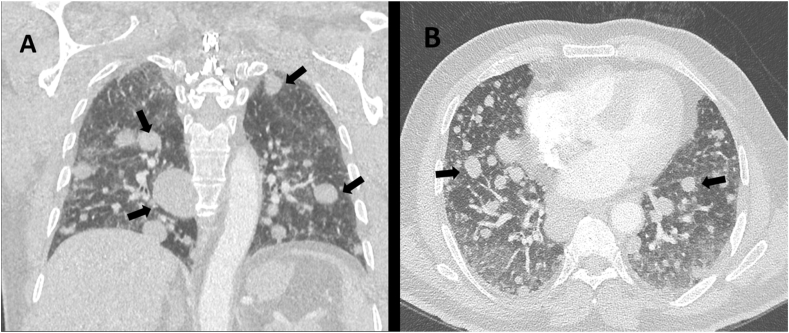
Computed Tomography scan of the thorax. A, coronal and B, axial planes showing multiple variable-sized solid pulmonary nodules (black arrows) at both lung parenchyma, in keeping with metastasis.
